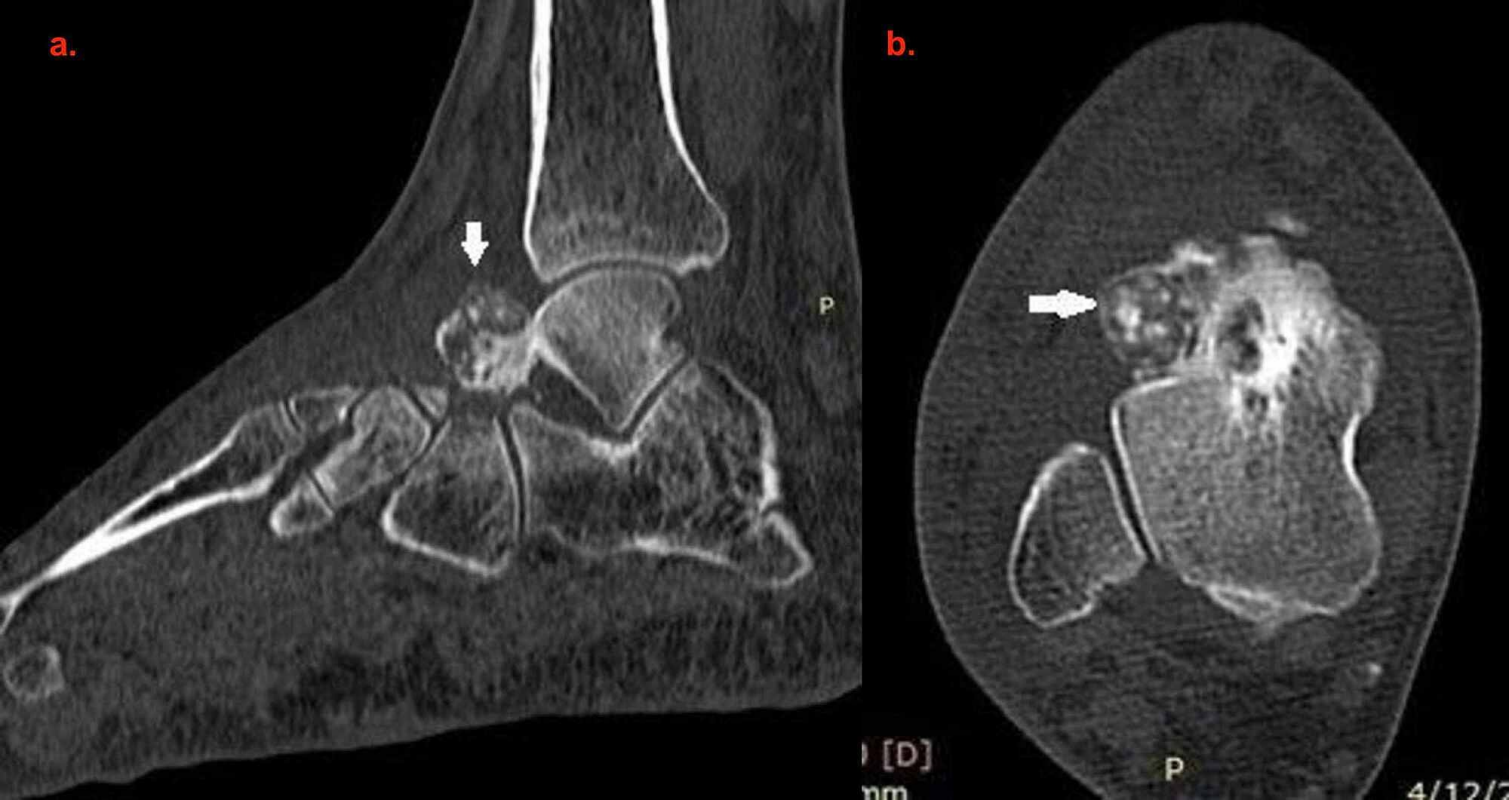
Sagittal reformatted (a) and axial (b) CT of the ankle and hindfoot demonstrates an exophytic capsulated osseous lesion at neck of talus having punctate calcifications (white arrow)
